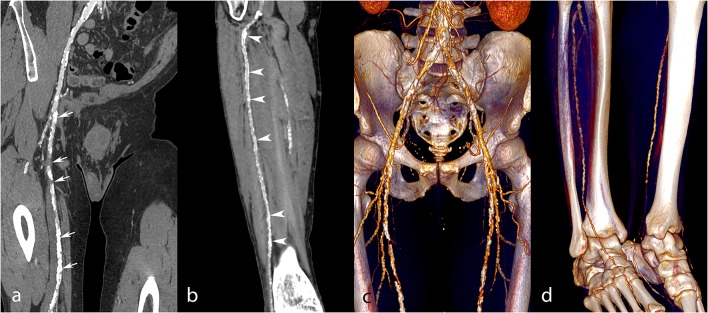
Lower extremity CT angiography obtained with the SA technique in a 54-year-old man with a history of insulin-requiring type 2 diabetes, hypertension, past stroke, and myocardial infarction, who was admitted due to Fontaine stage III peripheral arterial disease. Curvilinear reconstructions (a,b) and corresponding volume rendering reconstructions (c,d) of the right iliofemoral arterial system and anterior tibial artery. Several severe stenoses and occlusions were found along the superficial femoral artery (a,c, white arrows). At the tibial and pedal levels (b,d), the arterial enhancement is non-optimal but sufficient for a diagnosis. Nevertheless, note the extensive arterial wall calcifications of the anterior tibial artery (white arrowheads) related to diabetes, rendering the analysis difficult. Attenuation measurements were as follows: aortic level, 261 HU; iliac level, 271 HU (right), and 256 HU (left); femoral level, 191 HU (right), and 176 HU (left); tibial level 187 HU (right), and 172 HU (left); pedal level, 112 HU (right), and 107 (left). HU, Hounsfield units.
